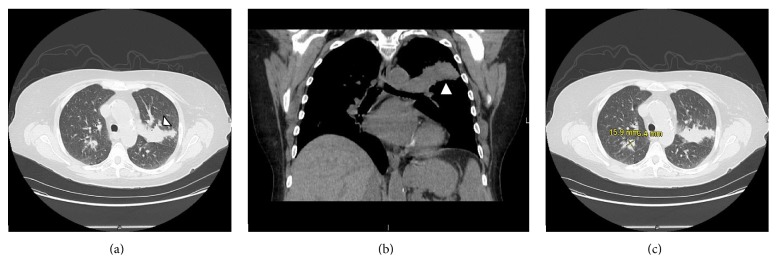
CT scan of the chest demonstrating lung lesions. (a) Transverse view CT scan without IV contrast shows a 3.6 by 4.9 by 2.9 cm mass in left upper lobe* (arrow)*. Interaction with the oblique fissure is noted. (b) Coronal view showing left upper lobe lesion* (arrow)* without any mediastinal or hilar lymphadenopathy. (c) A pulmonary nodule is visualized in the right lung (contralateral). This mass was not biopsied and was assumed to be secondary to the primary adenocarcinoma in the left lung.
